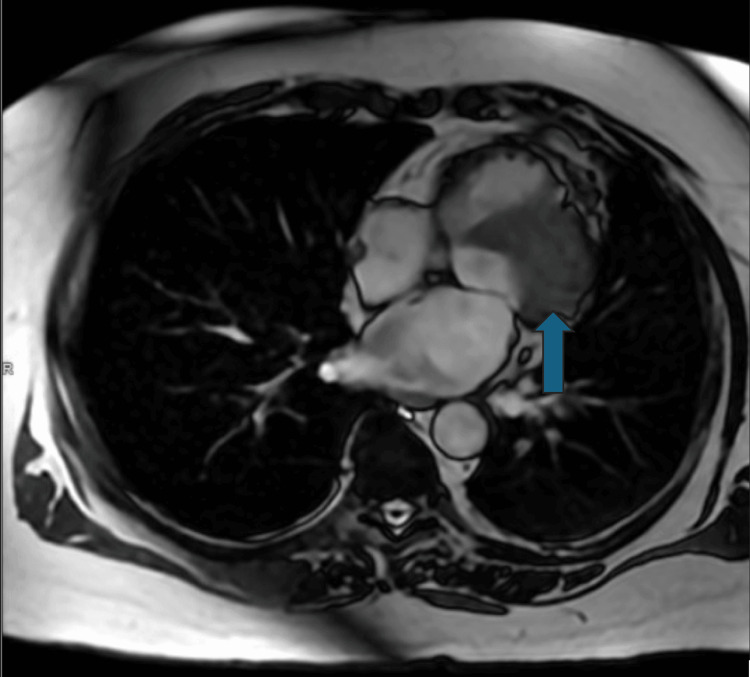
Cardiac MRI showing acute infarction. Arrow showing acute infarction.
